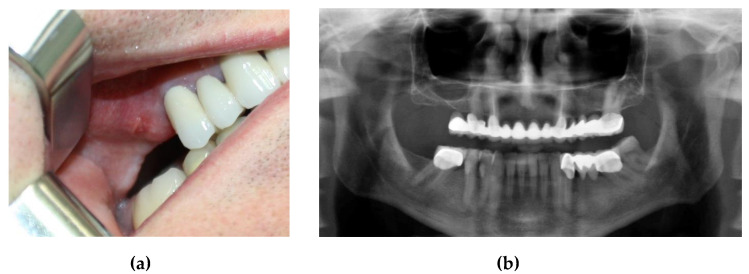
(a) Initial clinical assessment; (b) preoperative orthopantomogram (OPG) showing an insufficient height for implant insertion in the maxillary right first molar edentulous site.
